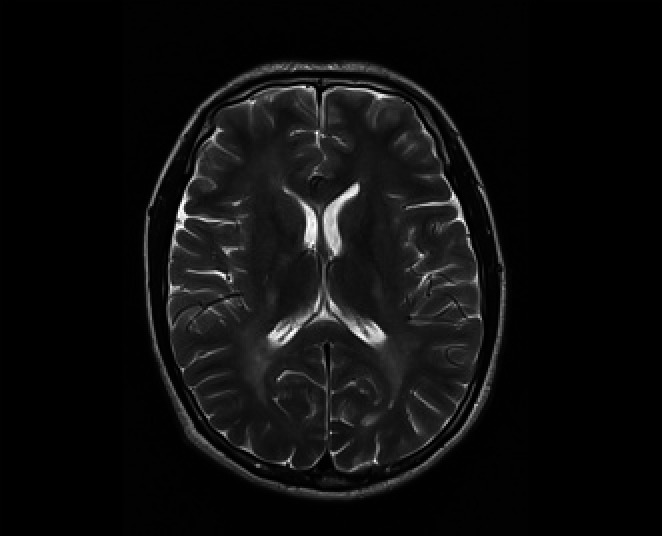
FIGURE 1 Left/Axial T2‐weighted MRI of the brain showing confluent hyperintense lesions in the parieto‐occipital deep white matter, extending through the splenium of the corpus callosum.
